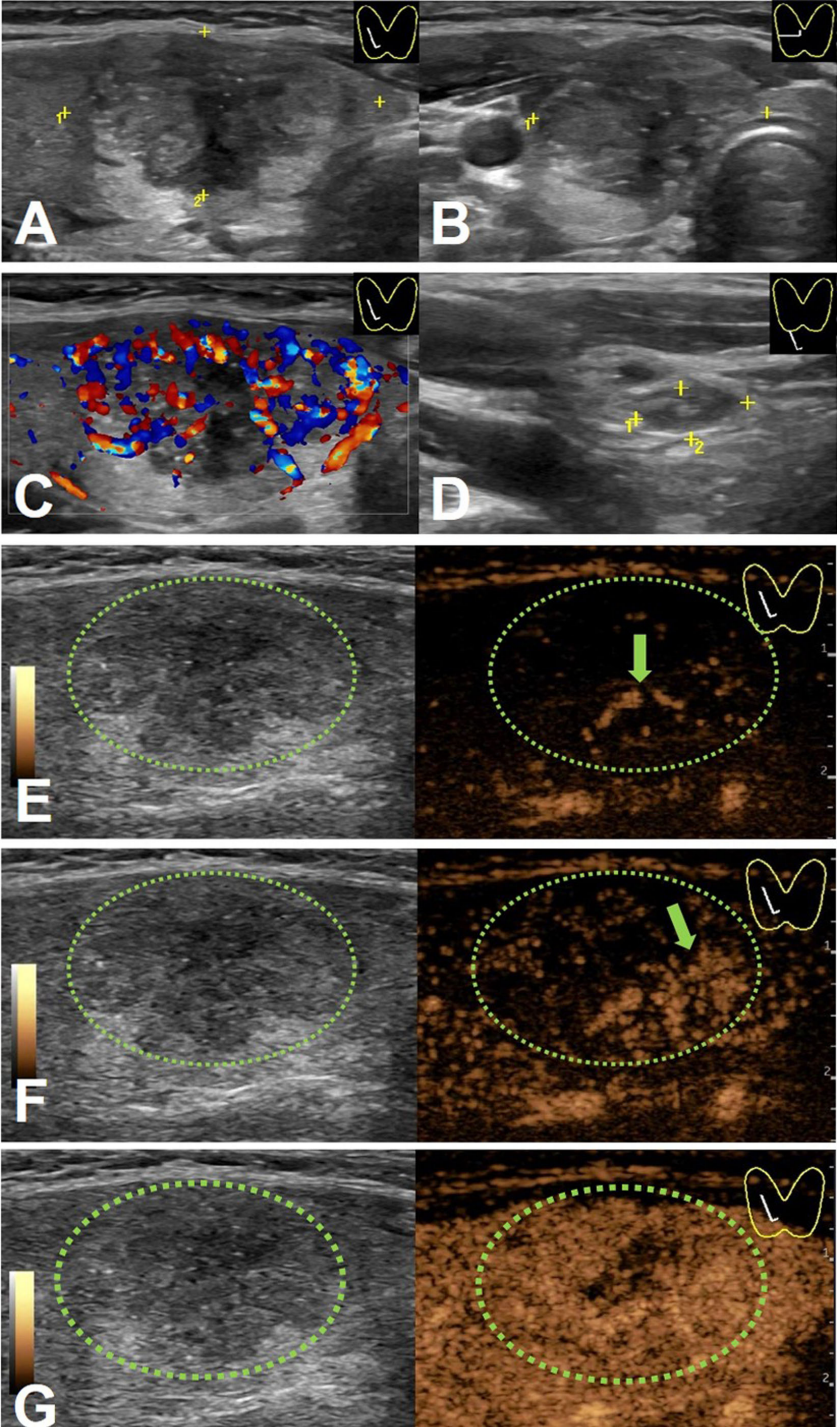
An 18-y-old female PTC patient with LN-prRLN metastasis. Initial longitudinal scan (A) and transverse scan (B) showing a 30-mm thyroid nodule with hypo-echogenicity, ill-defined margins, and petal calcification. (C) Doppler image revealing abundant signals in the nodule. (D) A level VI lymph node (yellow cross) identified on conventional US. (E) CEUS image 6 s after injection of contrast-enhanced agent, the thyroid nodule enhanced from the center to the periphery (green arrow). (F) CEUS image 7 s after injection of contrast-enhanced agent, the thyroid nodule showed heterogeneous enhancement (green arrow). (G) CEUS image 8 s after injection of contrast-enhanced agent, the thyroid nodule showed heterogeneous iso-enhancement.
